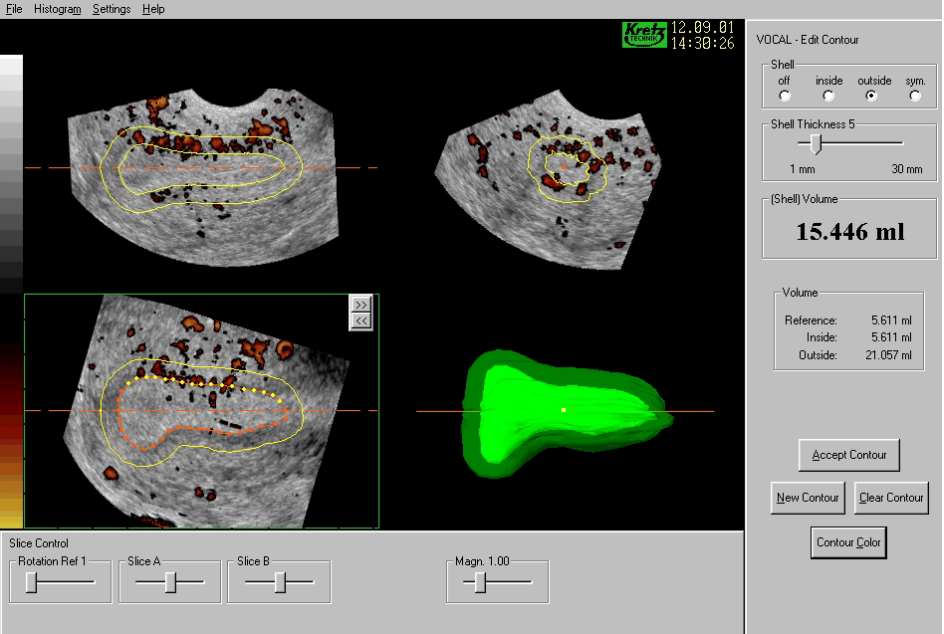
Three-dimensional power Doppler angiography of the uterine blood supply*. A three-dimensional dataset containing power Doppler information has been acquired from the uterus. VOCAL has then been used to define the myometrial-endometrial border and to apply a shell 5 mm outside of that contour to define the sub-endometrium, which can be clearly seen to be more vascular than the endometrium itself.
